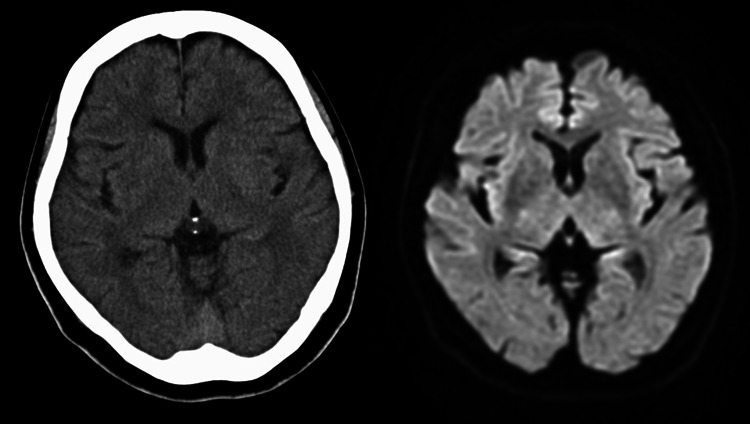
Head CT scan (left) at the ER showed no hemorrhagic lesions. MRI (right) showed neither fresh ischemic lesions nor abnormal signs of herpetic encephalitis
